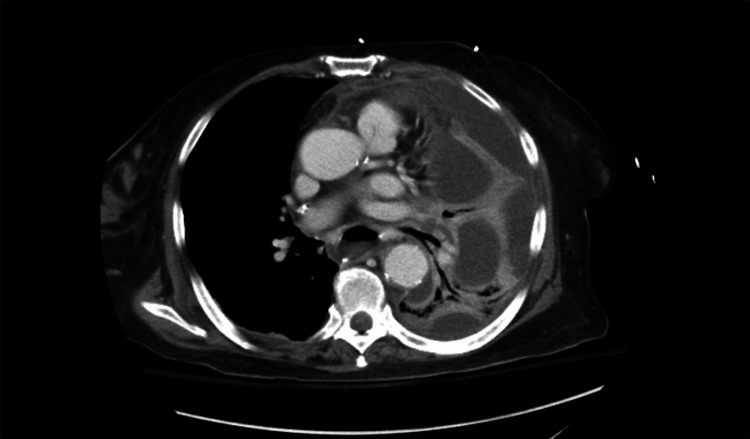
Chest computed tomography demonstrating a left-sided pleural effusion (arrow) with associated findings concerning for esophageal perforation, including adjacent mediastinal contamination. These findings correlate with the patient’s clinical presentation of chest pain and progression to septic shock.
